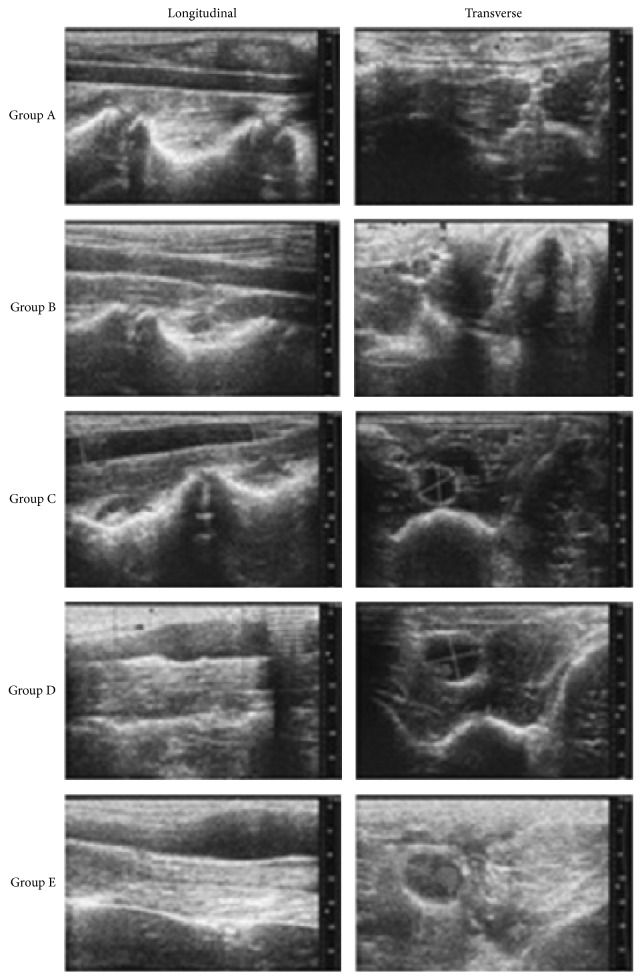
The diameter of the abdominal aorta differentially changed in response to different concentrations of elastase, as shown by Doppler ultrasound examinations of the abdominal aorta. The aneurysm diameter increased with an increasing concentration of elastase.
